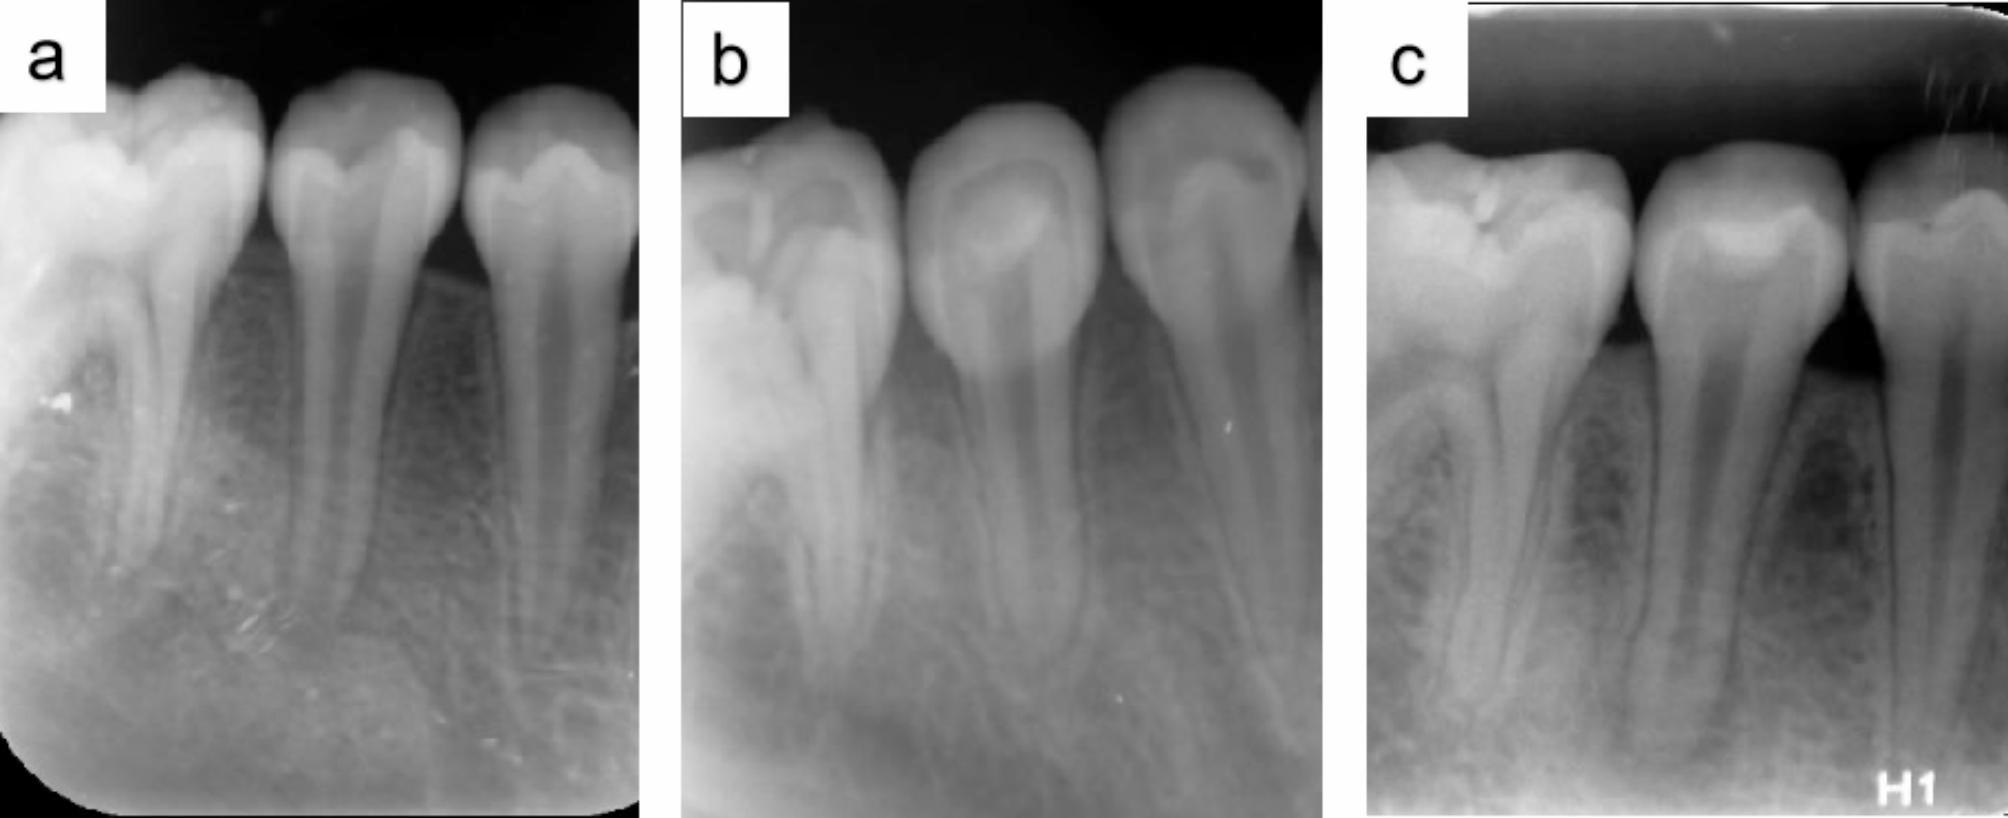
Periapical radiographs over the 60-month follow-up period. (a) Preoperative periapical radiograph of tooth 45 showing an incompletely developed root with a periapical lesion. (b) Postoperative periapical radiograph at the 18-month follow-up period after REPs showing resolution of the periapical lesion and healing of the periapical bone, with complete development of the root. (c) Postoperative periapical radiograph at the 60-month follow-up period showing a recurrence of the periapical lesion while the patient remained asymptomatic
